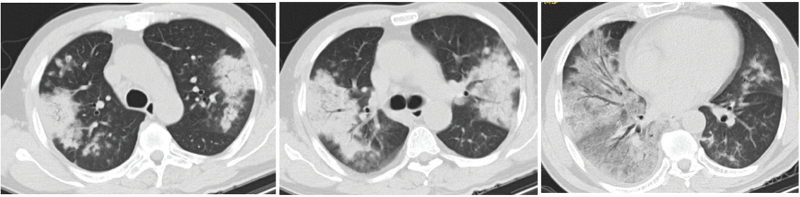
Chest computed tomography of Case 4, a 50-year-old male, showing multiple patchy, patchy and nodular consolidation shadows, ground-glass opacity, air-bronchogram, blurred edges, slightly thickened interlobular septa in both lungs, and a small right pleural effusion.
